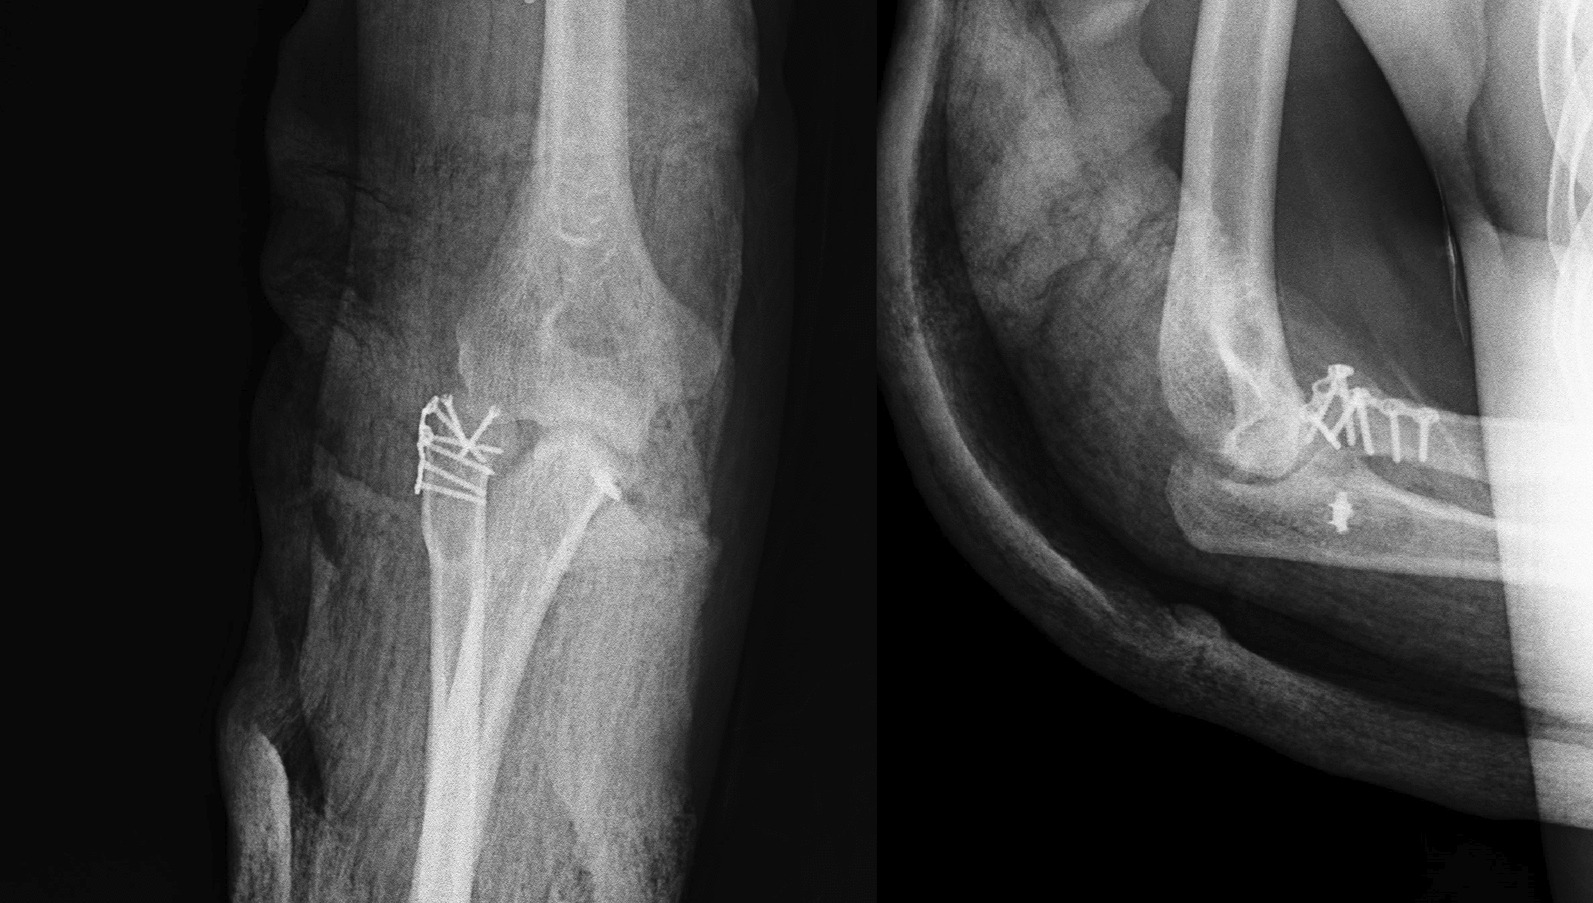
Postoperative right elbow X-rays
